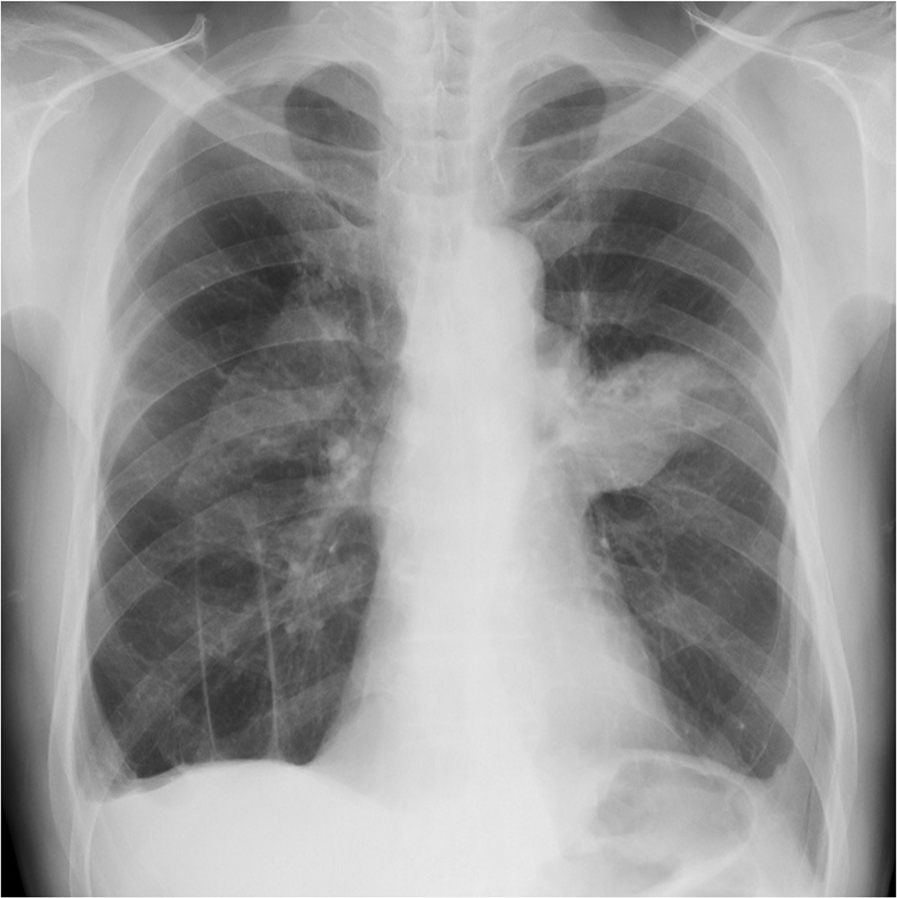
A chest X-ray revealed a mass shadow in the left lung field and a positive silhouette sign for the left second arch
